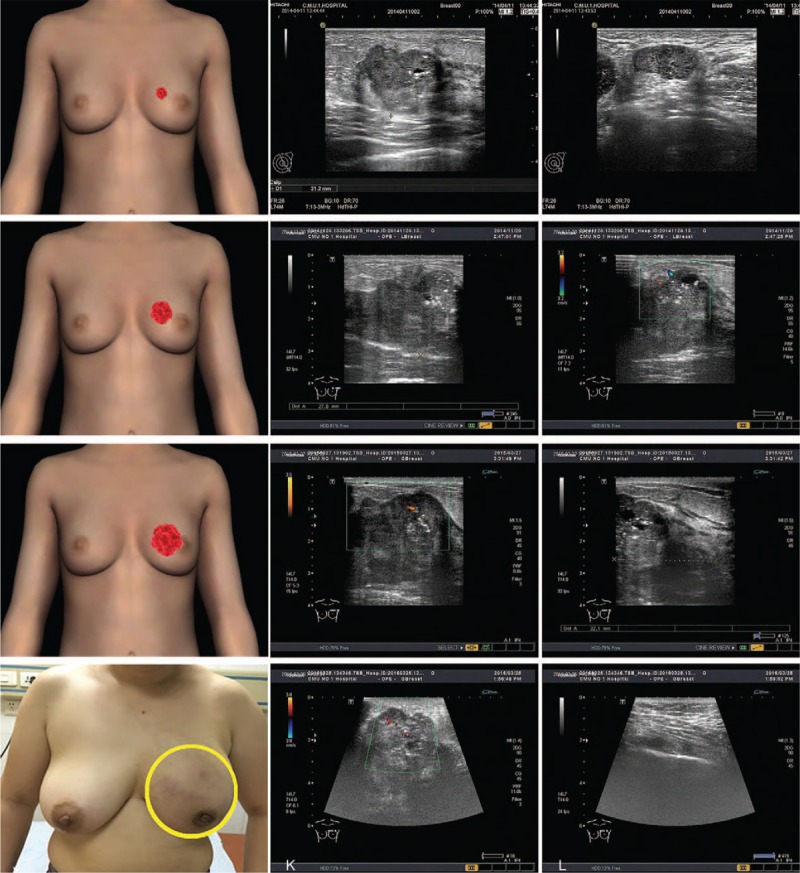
Tumor solid in this patient. (A) Tumor mass model on April 11, 2014. The tumor mass was 2.93 × 2.79 × 2.12 cm at 11 o’clock position, 2.6 cm from the nipple. (B and C) Tumor mass on ultrasound in April 11, 2014. (D) Tumor mass on November 20, 2014. It was 4.56 × 2.09 × 2.78 cm as detected by ultrasound. (E and F) Tumor mass on ultrasound on November 20, 2014. (G) Tumor mass model on March 27, 2015. It was 7.3 × 6.0 × 4.0 cm as detected by ultrasound. (H and I) Tumor mass on ultrasound on March 27, 2015. (J) Tumor mass in this patient on March 28, 2016. The size of the tumor was 16 × 13.5 × 12 cm, which occupied the whole left breast with skin implanted and nipple abnormality. (K and L) Tumor mass on ultrasound on March 25, 2016.
